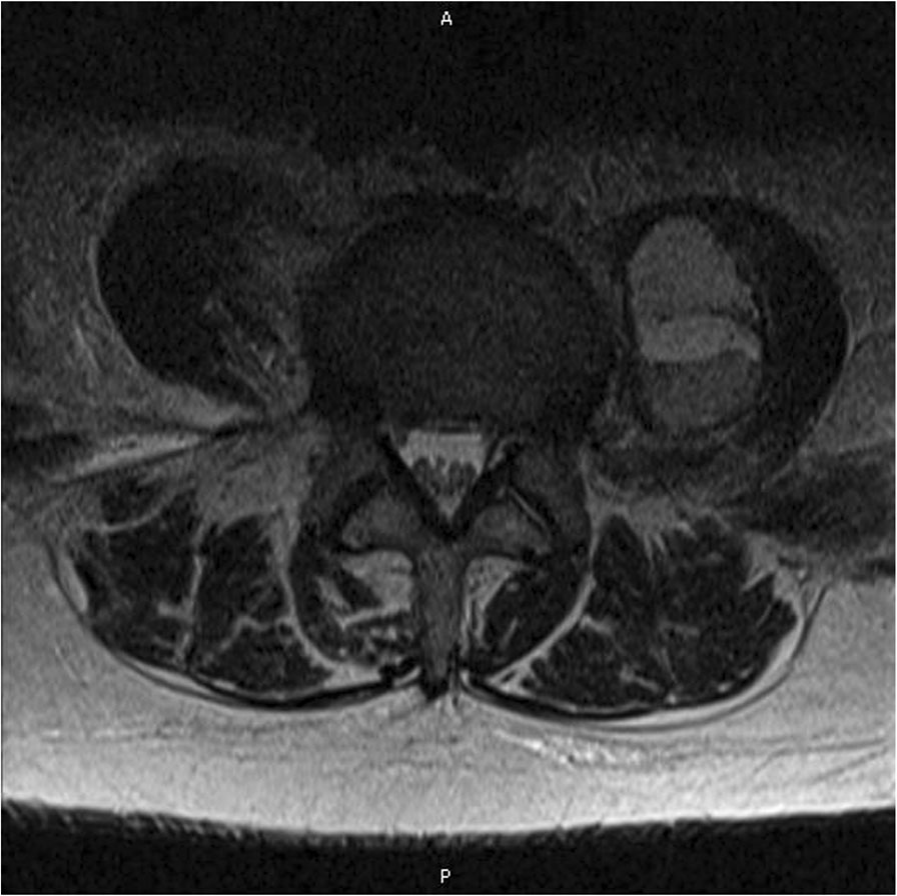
Magnetic resonance imaging showing the left psoas hematoma
